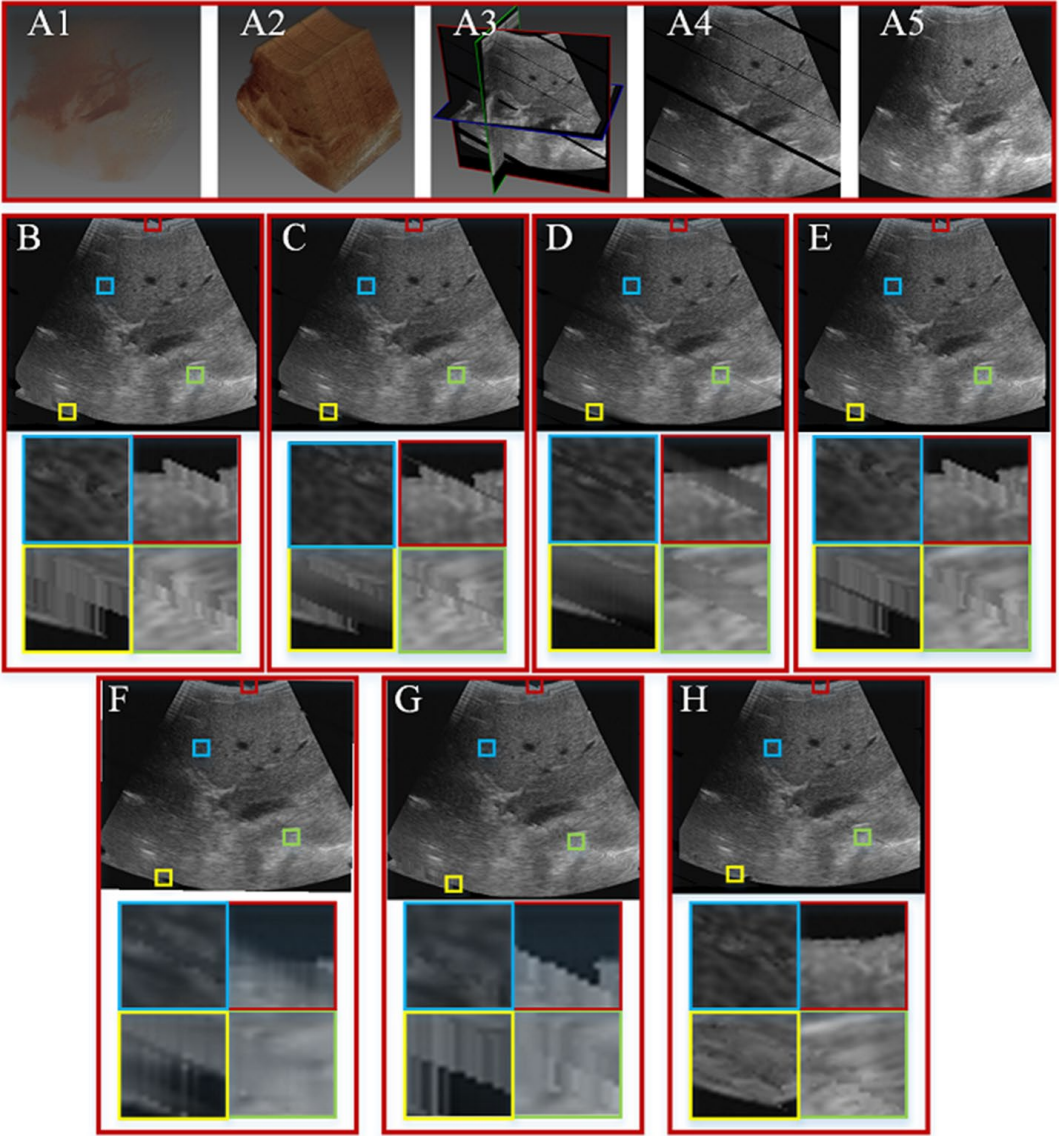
Comparison of different reconstruction results for data 2. A1 Volume rendering of the ultrasound data. A2 Ultrasound volume with vacant patches. A3 Cross-sections of the ultrasound volume data. A4 Ultrasound slice with vacant patches. A5 Original ultrasound slice. B–H Reconstruction results of the VNN, PNN, DW, FMM, KR, BI and GPM methods. The third column shows the magnified regions of interest corresponding to the second column
